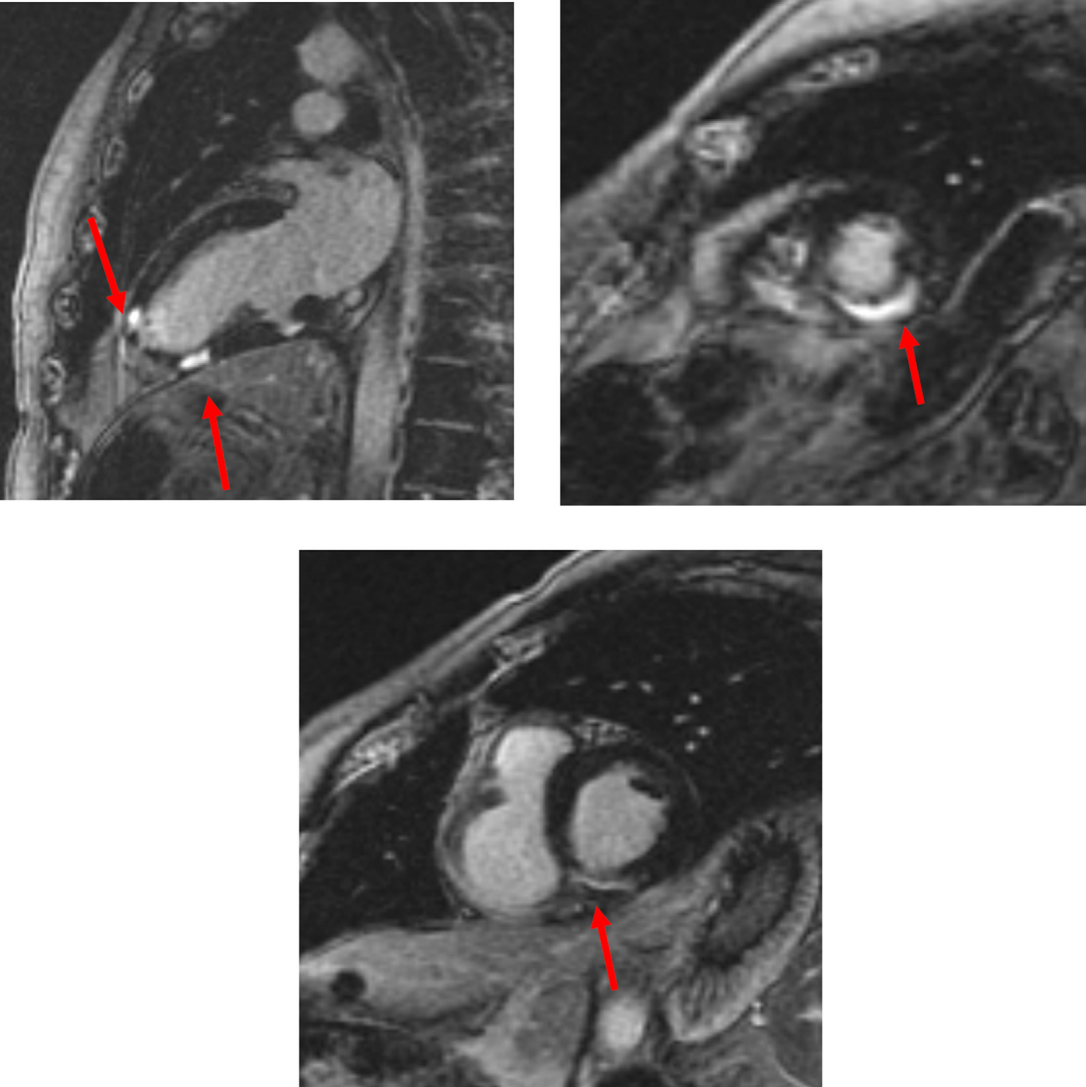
CMR Showing Lymphoma Involving the Myocardium in Patient 1 Cardiac magnetic resonance (CMR) shows patchy regions of mid myocardial/transmural foci of delayed enhancement involving the anterior, septal and inferior walls of the left ventricle (red arrows), corresponding to site of lymphomatous involvement on positron emission tomography–computed tomography imaging.
